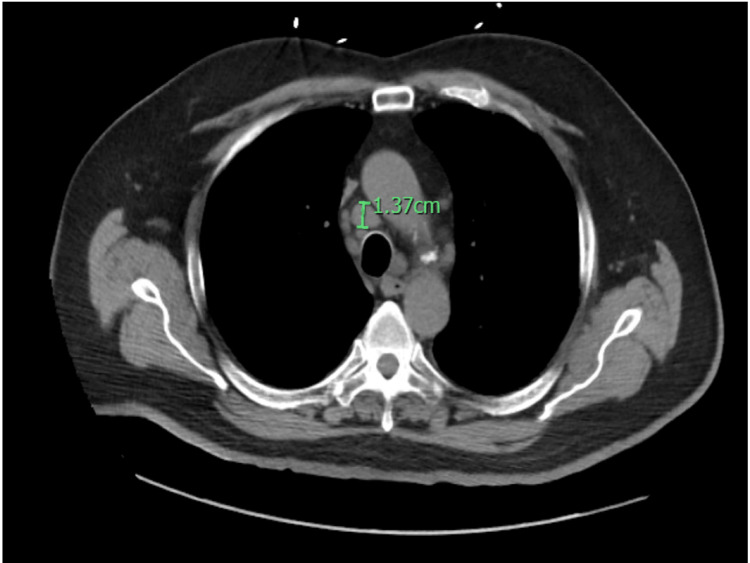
CT chest without contrast (axial view) showed the enlarged right peritracheal lymph node measuring 1.37 cm.
